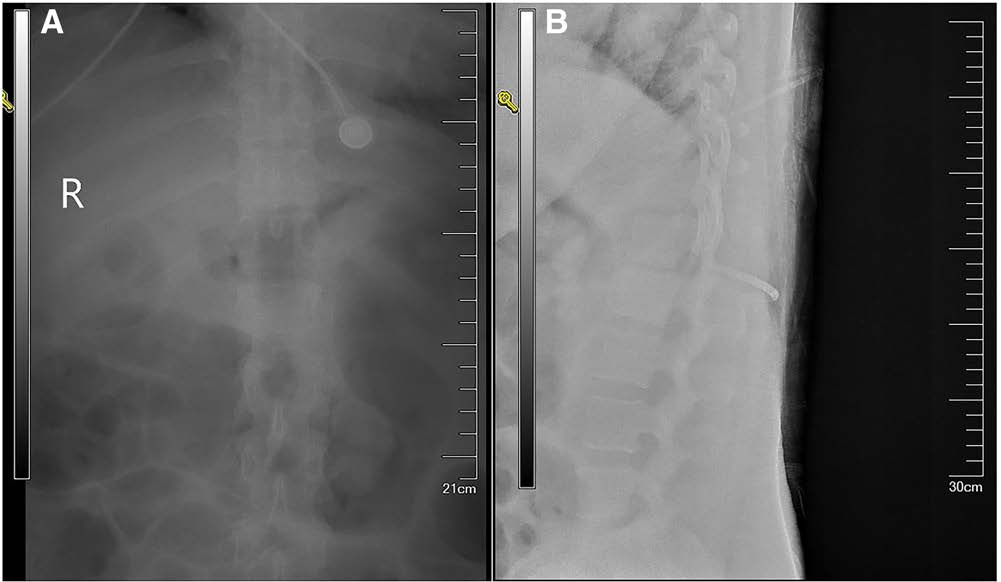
(A) Orthopantomogram of the lumbar spine; (B) lateral disk of the lumbar spine.
